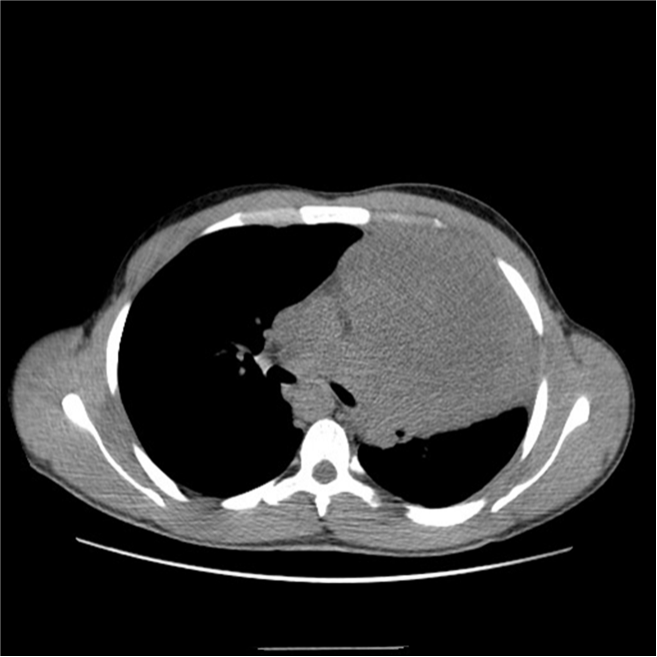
Computed tomography scan of the chest: a huge tumor of mediastinum before treatment.
